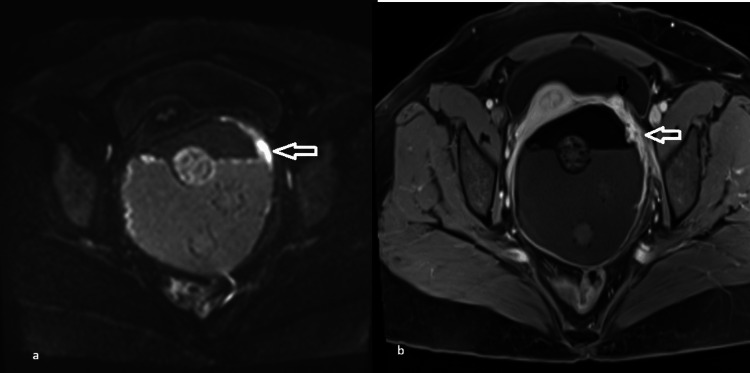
Enhancing mural thickening with diffusion restriction on MRI MRI: magnetic resonance imaging (a) Diffusion-weighted image (b = 1400 s/mm²) demonstrating focal mural thickening along the cyst wall with corresponding hyperintense signal indicating restricted diffusion (white arrow). (b) Axial T1-weighted fat-saturated postcontrast image showing subtle enhancing wall thickening (white arrow) along the cyst wall, with adjacent residual enhancing ovarian parenchyma (black arrow)
